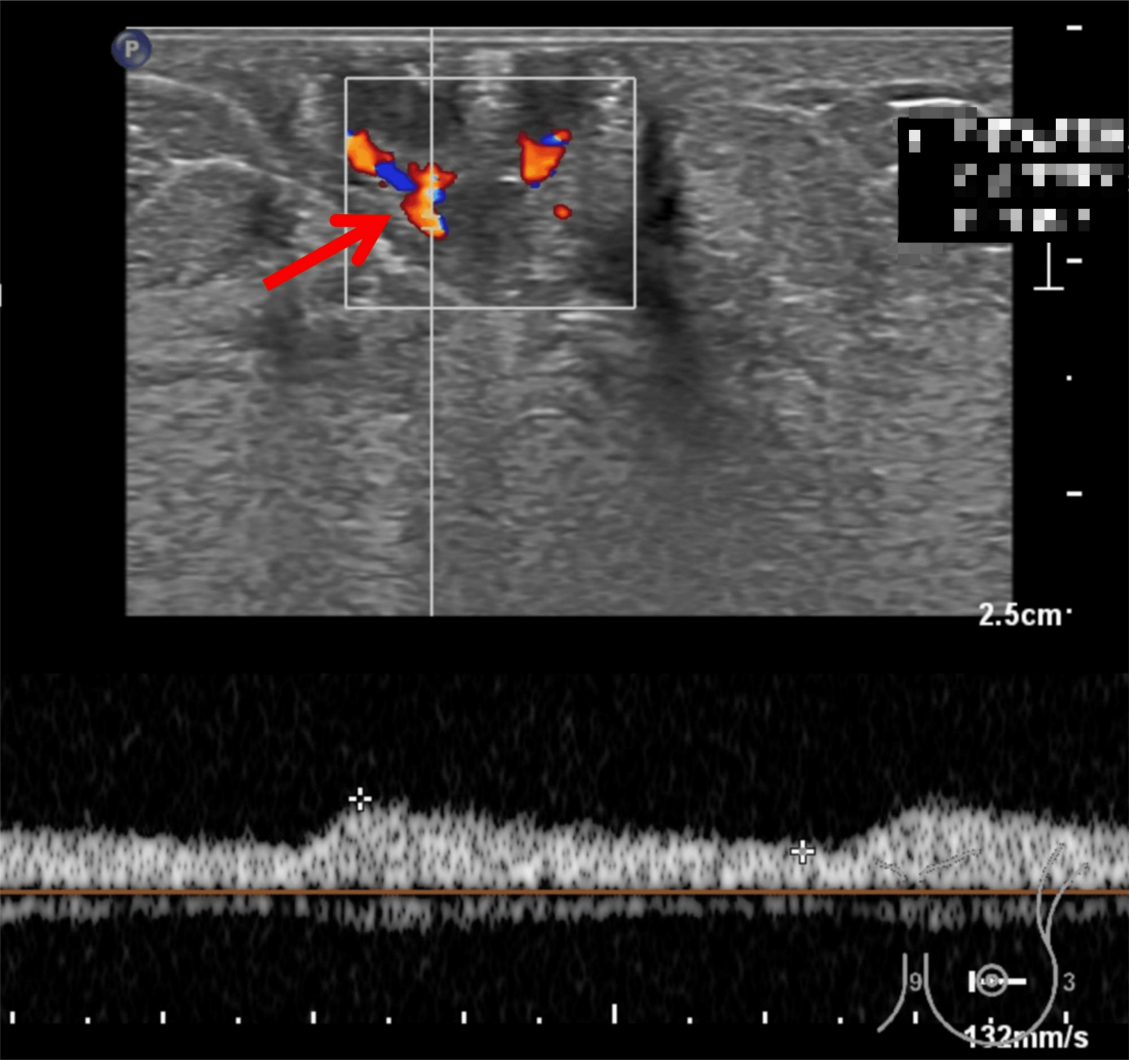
Ultrasonographic Findings of the Left Breast (B) Color Doppler ultrasound showing punctate blood flow signals within the hypoechoic lesion. Spectral Doppler analysis reveals a peak systolic velocity of 8.10 cm/s, an end-diastolic velocity of 3.53 cm/s, and a resistance index (RI) of 0.56, indicating intralesional vascularity.
